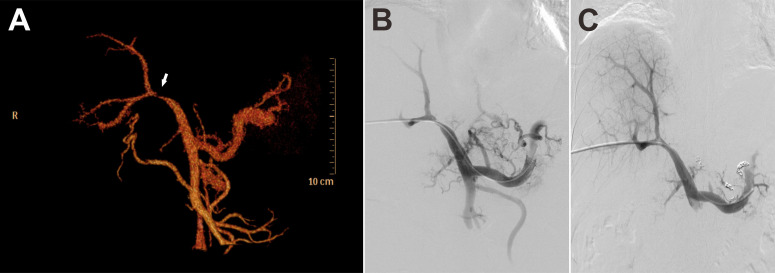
CT angiography (CTA) with three-dimensional reconstruction of portal system demonstrated severe stenosis of main portal vein (A, arrow). Portal vein stenosis (B) was relieved with portal venous angioplasty by balloon dilation (C).
